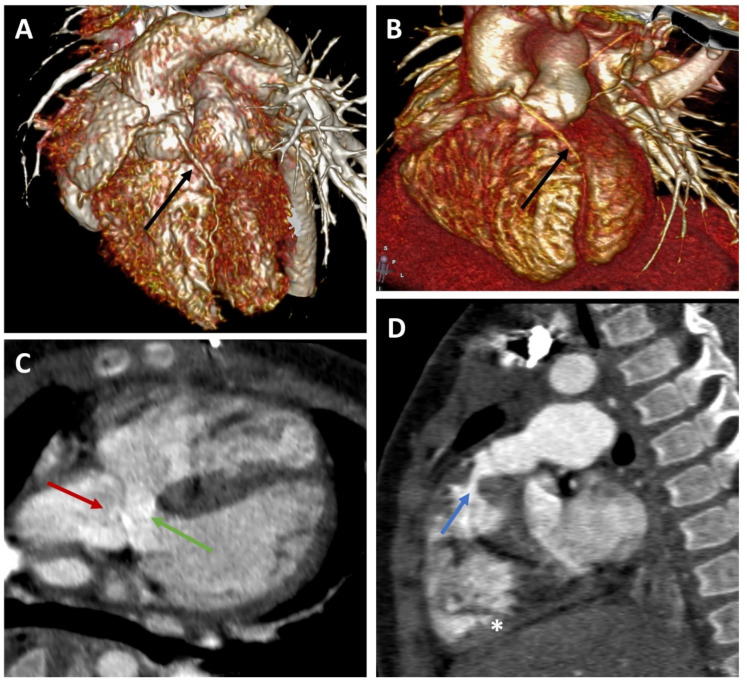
Representative CT angiography images. (A,B) Volume-rendering technique (VRT) reconstructions for CT images of 2 patients with Fallot tetralogy (ages: 7 and 14 months). Black arrow: anomalous course of the left anterior descending artery over the right ventricle outflow tract (RVOT). (C,D) Double oblique reconstruction of CT images in an infant with Fallot tetralogy. Red arrow: Mildly dilated aortic root overlapping the interventricular septum. Green arrow: Ventricular septal defect. Blue arrow: Narrowing of the right ventricular outflow tract (subpulmonary stenosis). The right ventricle (star) is mildly hypertrophied.
